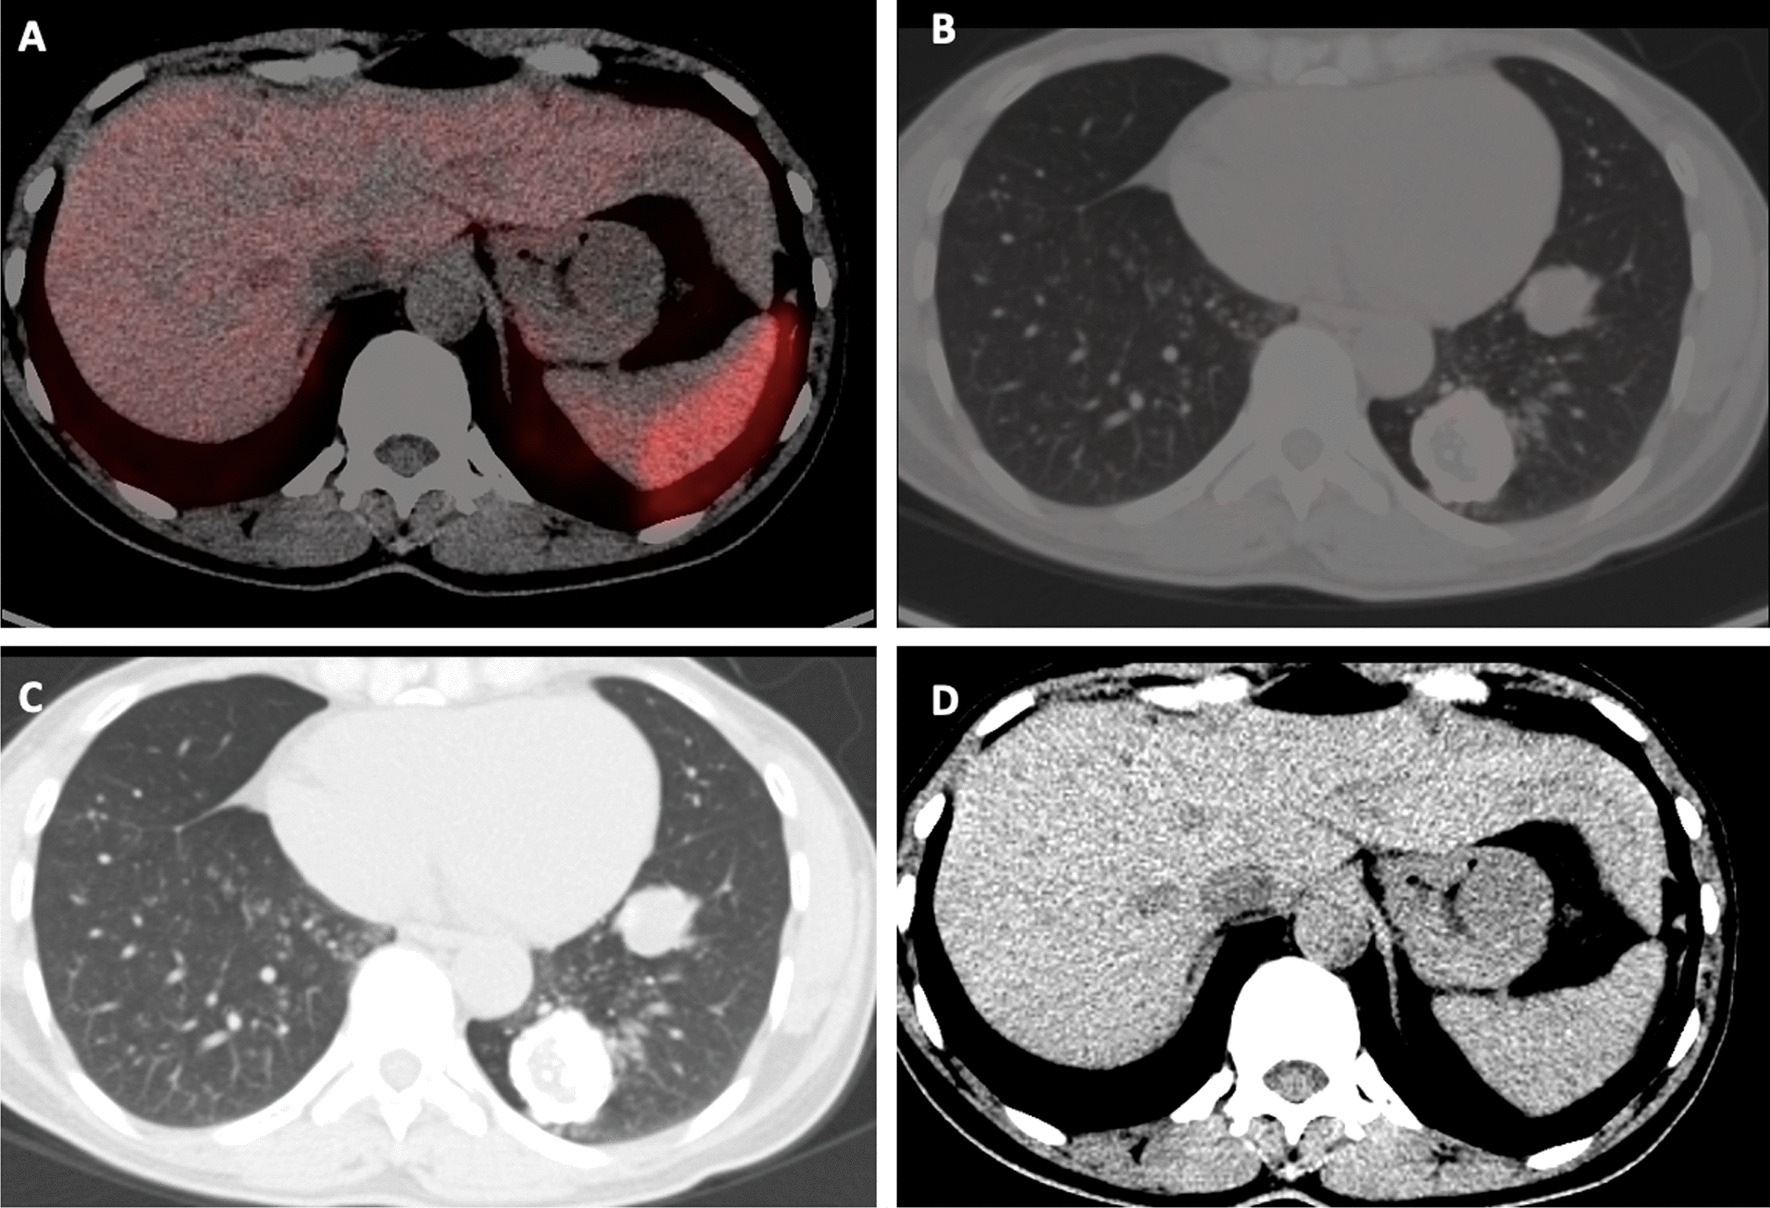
A Axial CT section abdomen showing endo-exophytic lesion from gastric fundus showing no significant tracer uptake on fused PET-CT (B). C Axial CT thorax showing soft tissue density nodular lesions in left lung lower lobe with no significant tracer uptake on fused PET-CT (D)
